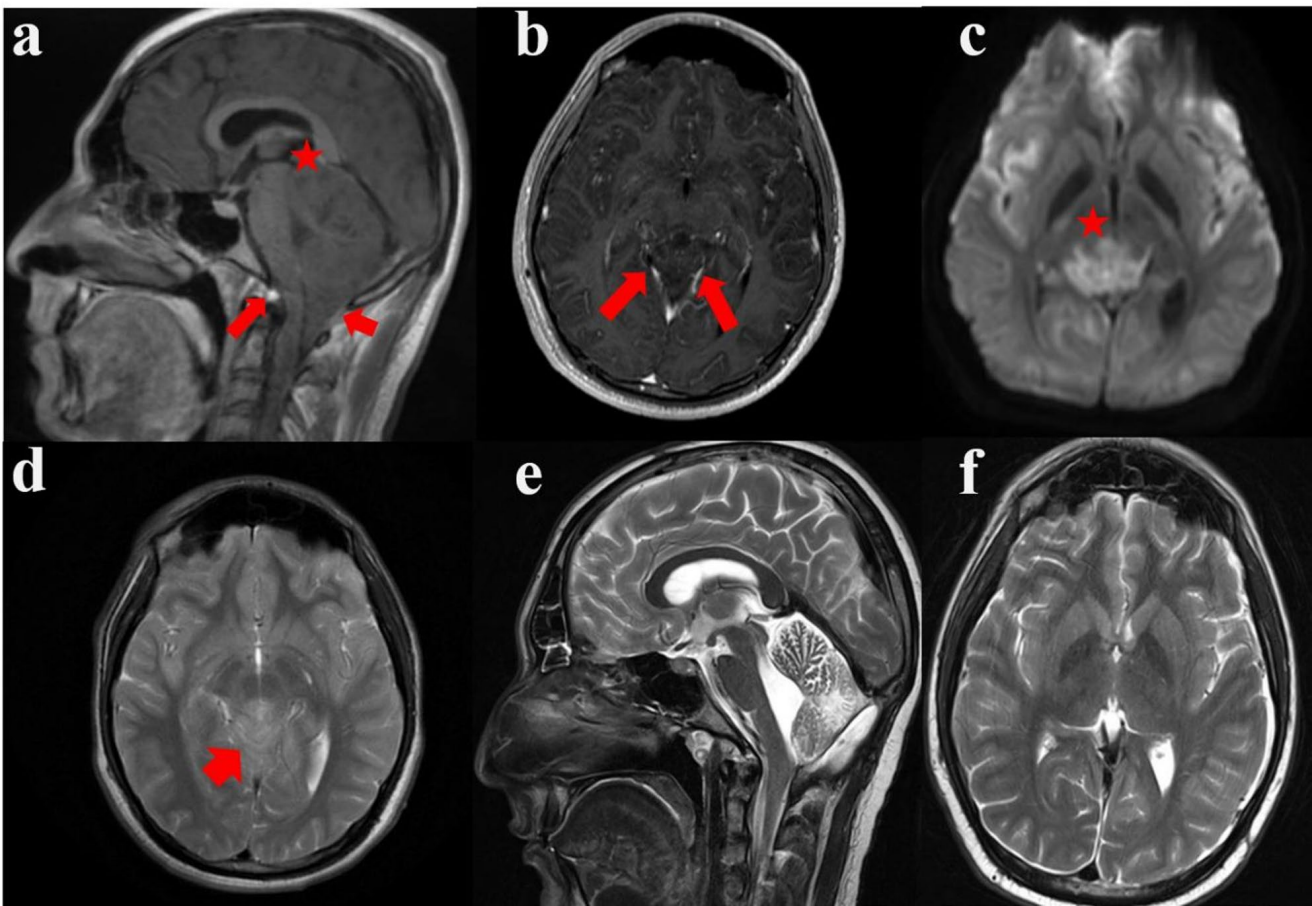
Preoperative and postoperative radiological findings. a T1W sagittal MRI reveals cerebellar tonsillar herniation along with caudal herniation of particular portions of the brainstem (often obex of the medulla oblangata) through the foramen magnum; Chiari type 1.5 malformation. b T1W axial gadolinium contrast enhanced MR imaging reveals 3 cm in diameter weakly heterogeneous enhancing mass in the pineal gland area compressing deep brain veins. c DWI axial MRI reveals diffusion rectricted areas mass lesion, originating from the pineal area extending to the mesencephalon d T2W axial MRI reveals hyperintense mass lesion, originating from the pineal area, infiltrating the superior cerebellar peduncles, extending to the mesencephalon and aqueductal region, and narrowing the aqueduct e T2W sagittal MRI decompressing of deep cerebral veins due to total resection of tumoral lesion and craniocervical junction due to bilateral cerebellary tonsillectomy and craniectomy f T2W axial MRI reveals decompressing of deep cerebral veins due to total resection of tumoral lesion. Red asterix indicates a tumour mass
